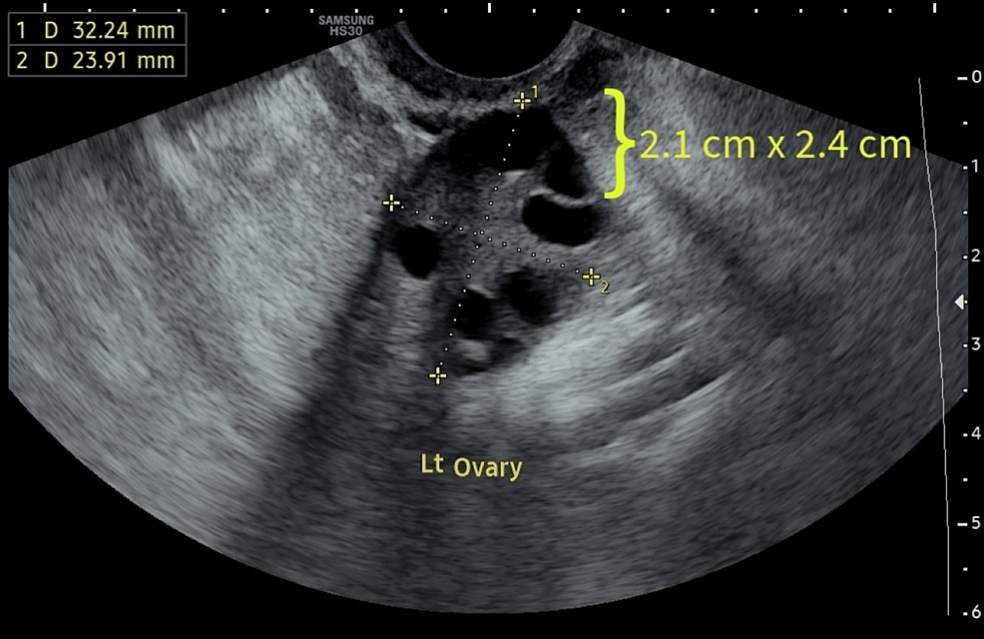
Day 2 transvaginal sonography of the patient's left ovary
